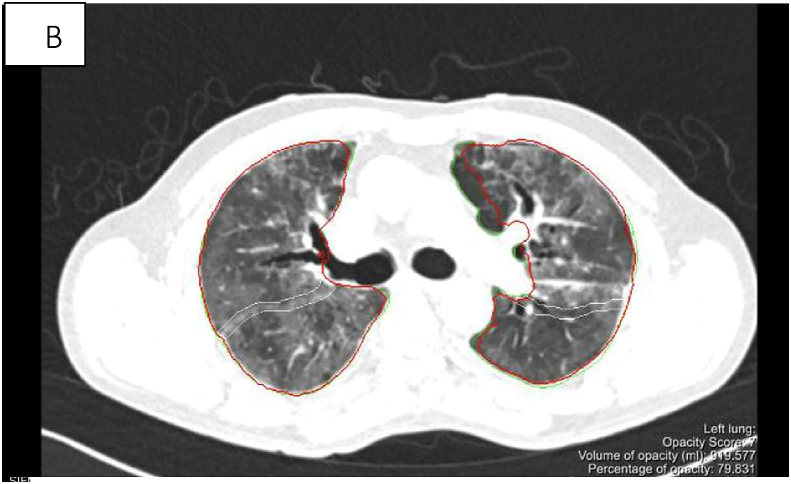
HRCT Chest. Resolution of previously noted pneumothorax. Bilateral ground glass changes.
